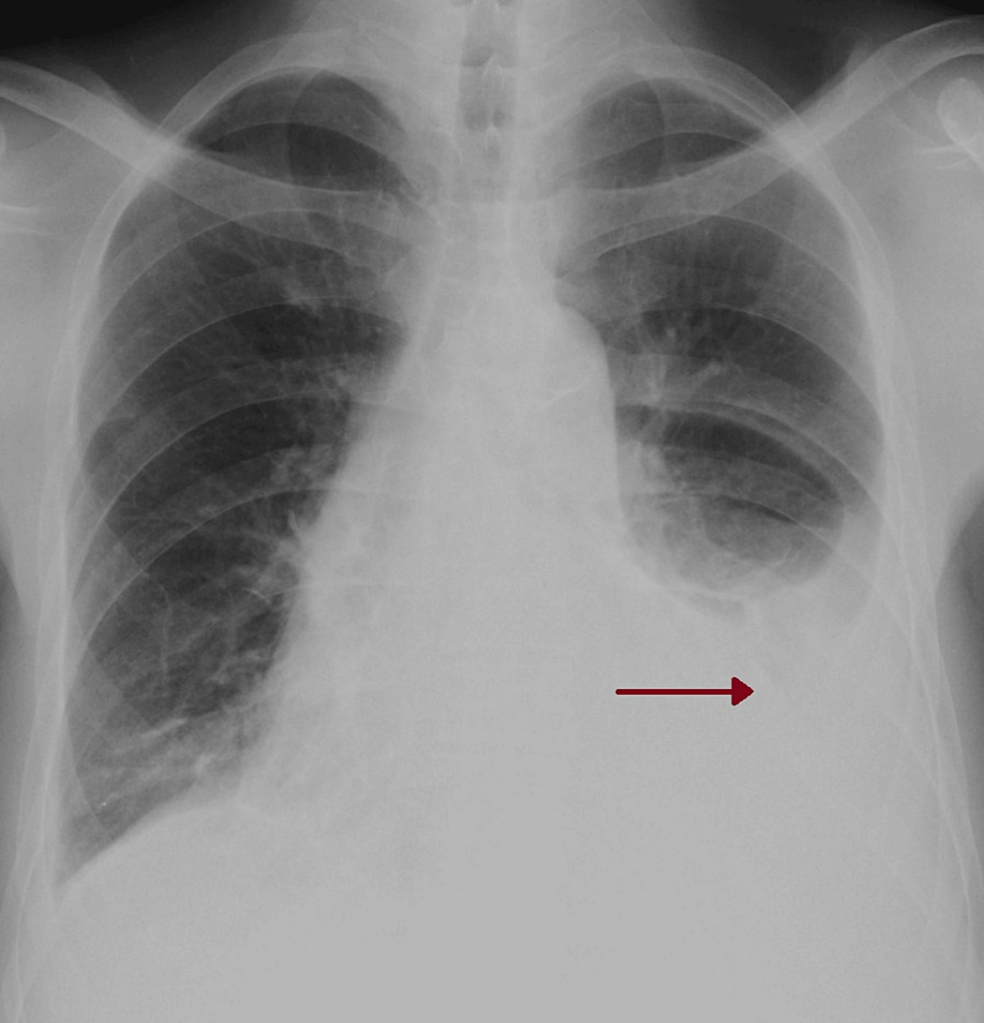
First chest x-ray at a hemodialysis clinic Red arrow: pleural effusion
